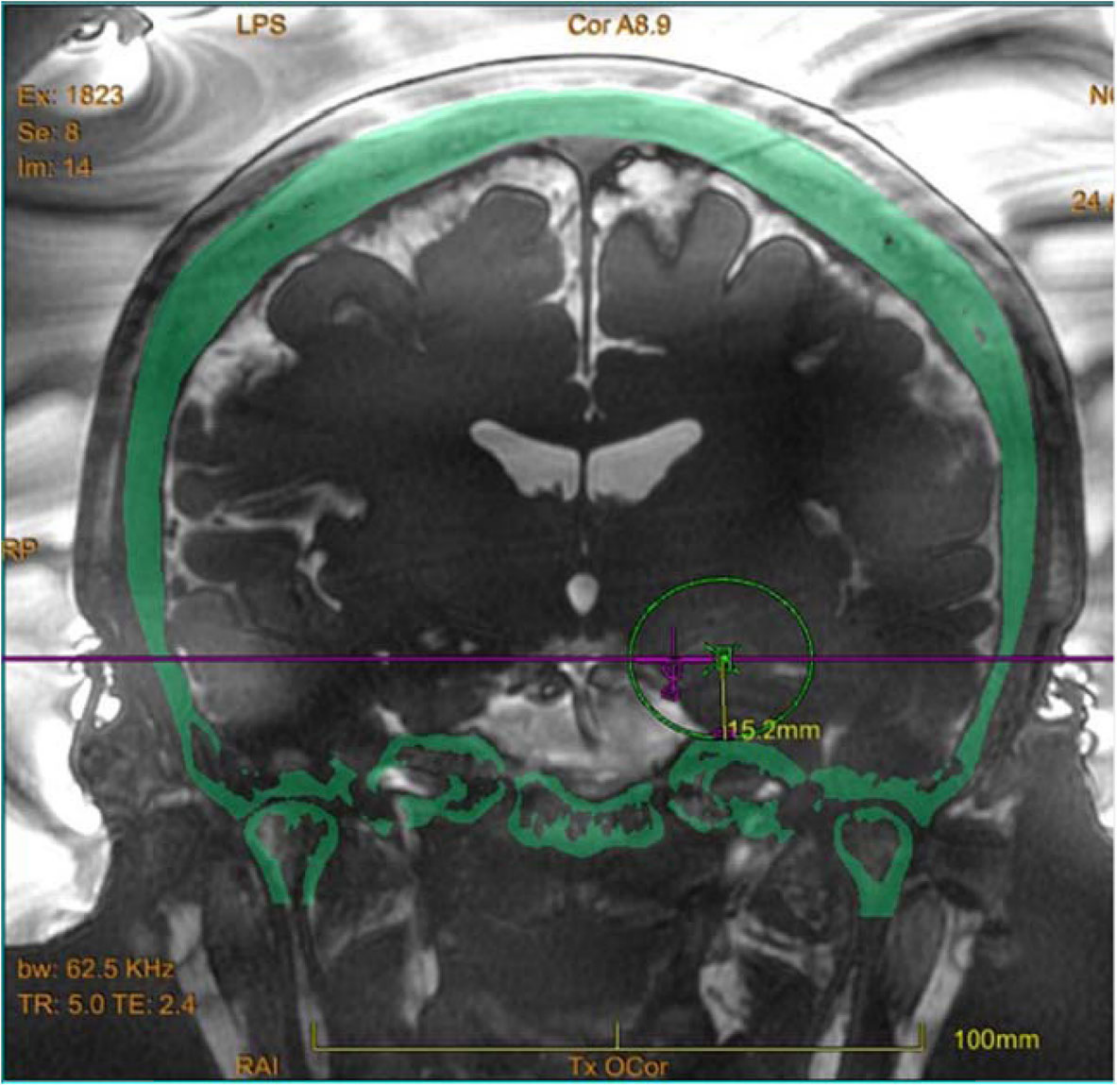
Live magnetic resonance imaging (MRI)
